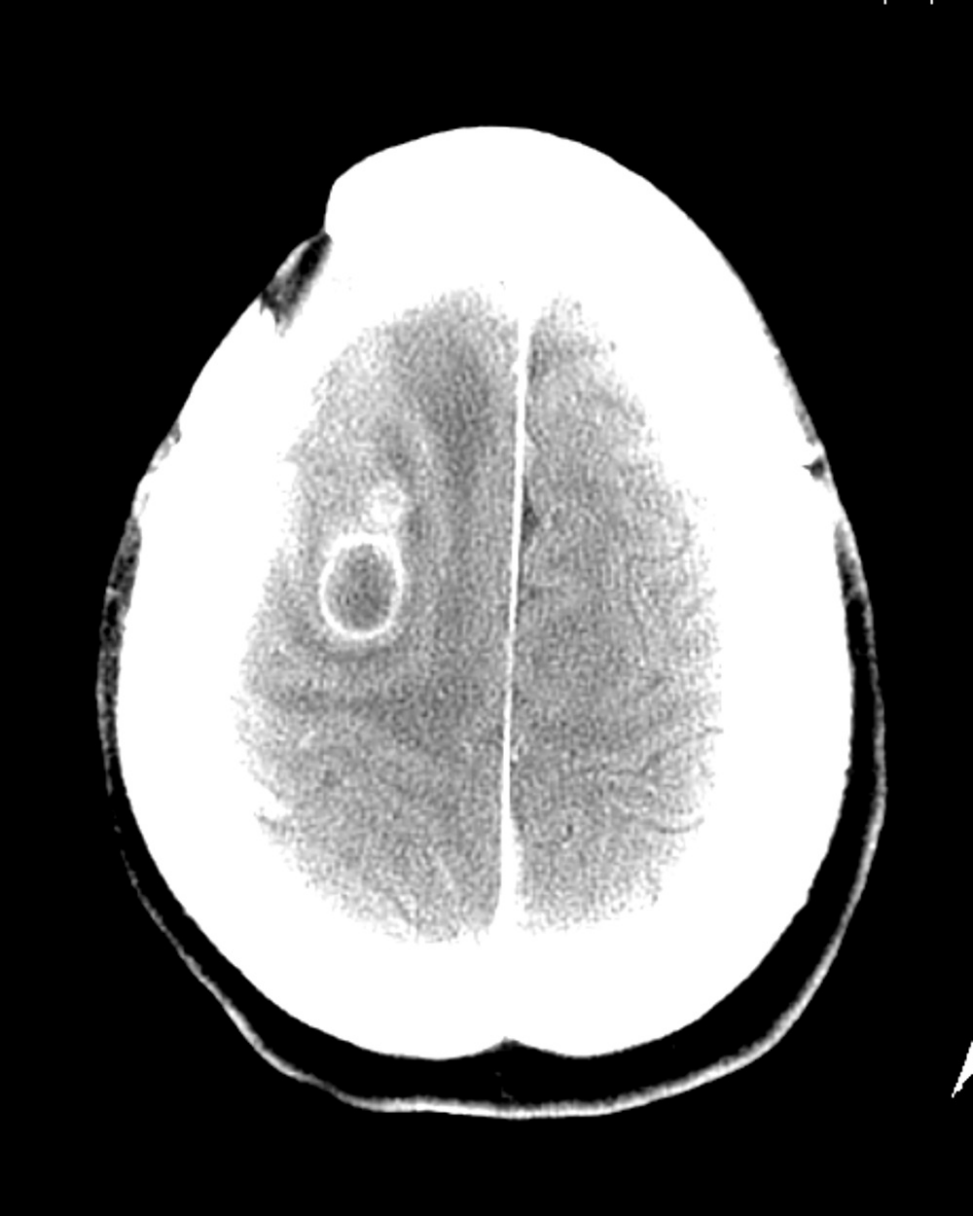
CT head with contrast showing right frontal intraparenchymal brain abscess formation
